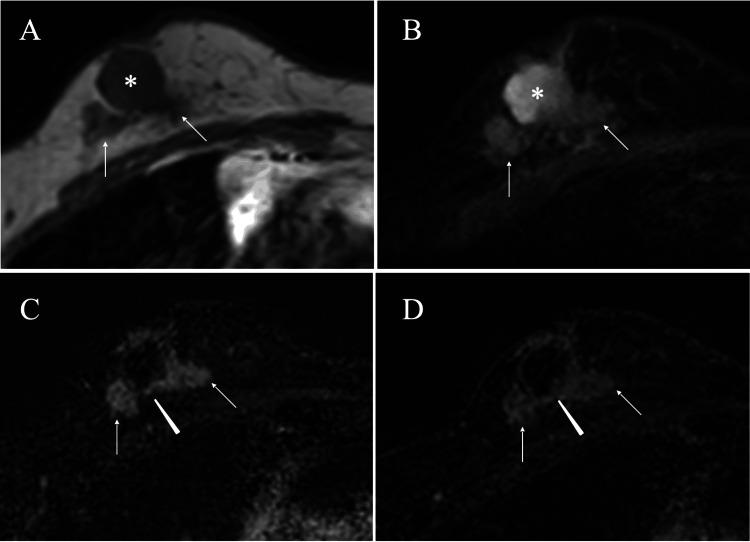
Magnetic Resonance Imaging (MRI) Findings MRI of the solid parts in the two masses showed low signals on T1-weighted images (A, arrows) and weak high signals on T2-weighted images (B, arrows). MRI of the cystic parts showed low signals on T1-weighted images (A, asterisk) and high signals on T2-weighted images (B, asterisk). Time-signal intensity images showed early enhancement on early phase images (C, arrows), retained weak enhancement on late phase images (D, arrows), and connection of the two masses at their deep borders (C and D, arrowhead).
